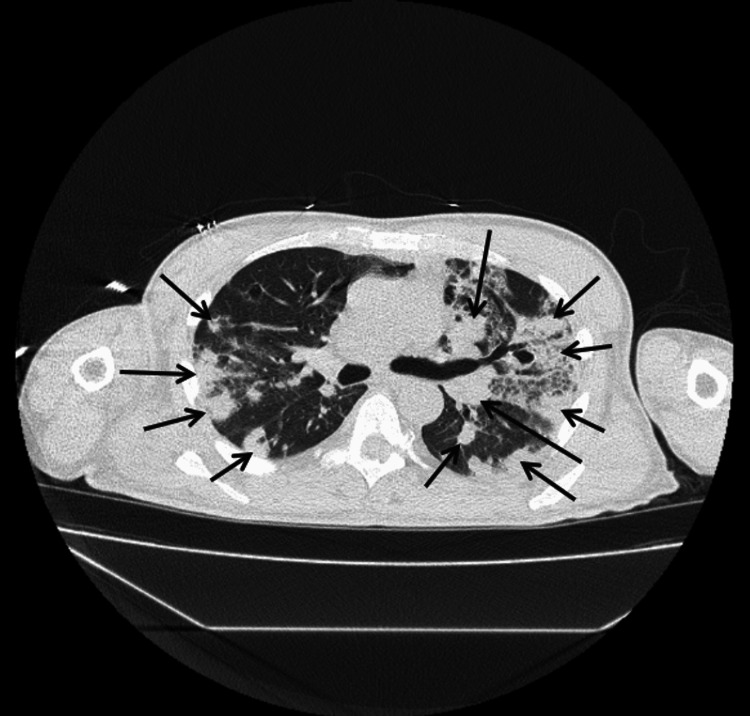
CT chest axial view without contrast showing the lower-middle parts of both lungs. The arrows show multiple, bilateral diffuse airspace cavitations with air bronchograms in some cavities, consistent with septic pulmonary emboli.
